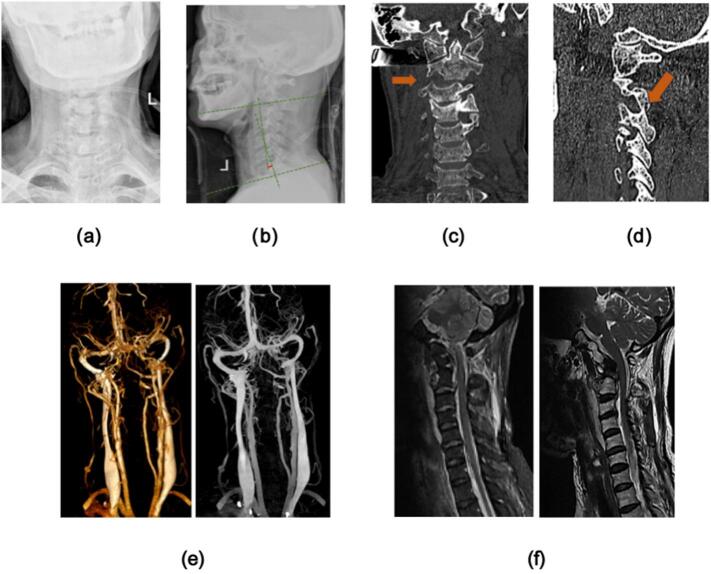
Preoperative cervical X-ray (2a. 2b), CT (2c, 2d), neck arterial-venous CTA (2e), and MRI (2f) were performed showing C2-C3 transverse dislocation and facet joint locked, the arterial-venous of the neck was no obvious injury (2e), and the Cobb angle of C2–7 cervical lordosis was measured to be 11°. MRI sagittal T2W1 showed C2–3 disc injury (2f).
